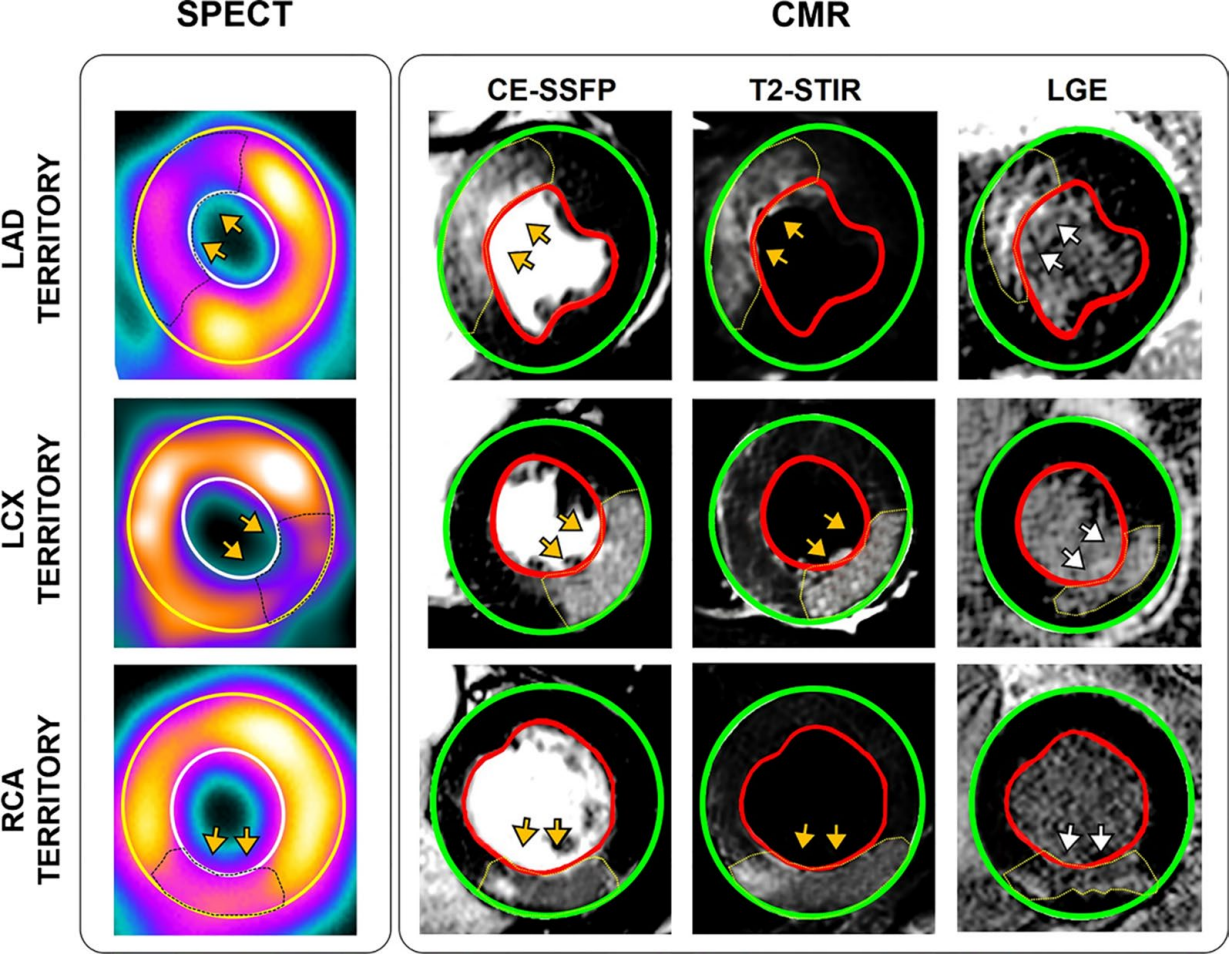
SPECT Validation in Acute MI Patient with LAD, LCx and RCA Myocardial Infarctions. The AAR determined using SPECT, CE-SSFP, T2-STIR, as well as infarct size determined using LGE images are shown from representative LAD, LCx and RCA myocardial infarctions. Yellow arrows demarcate the at-risk myocardium and the white arrows point to the infarct zone
